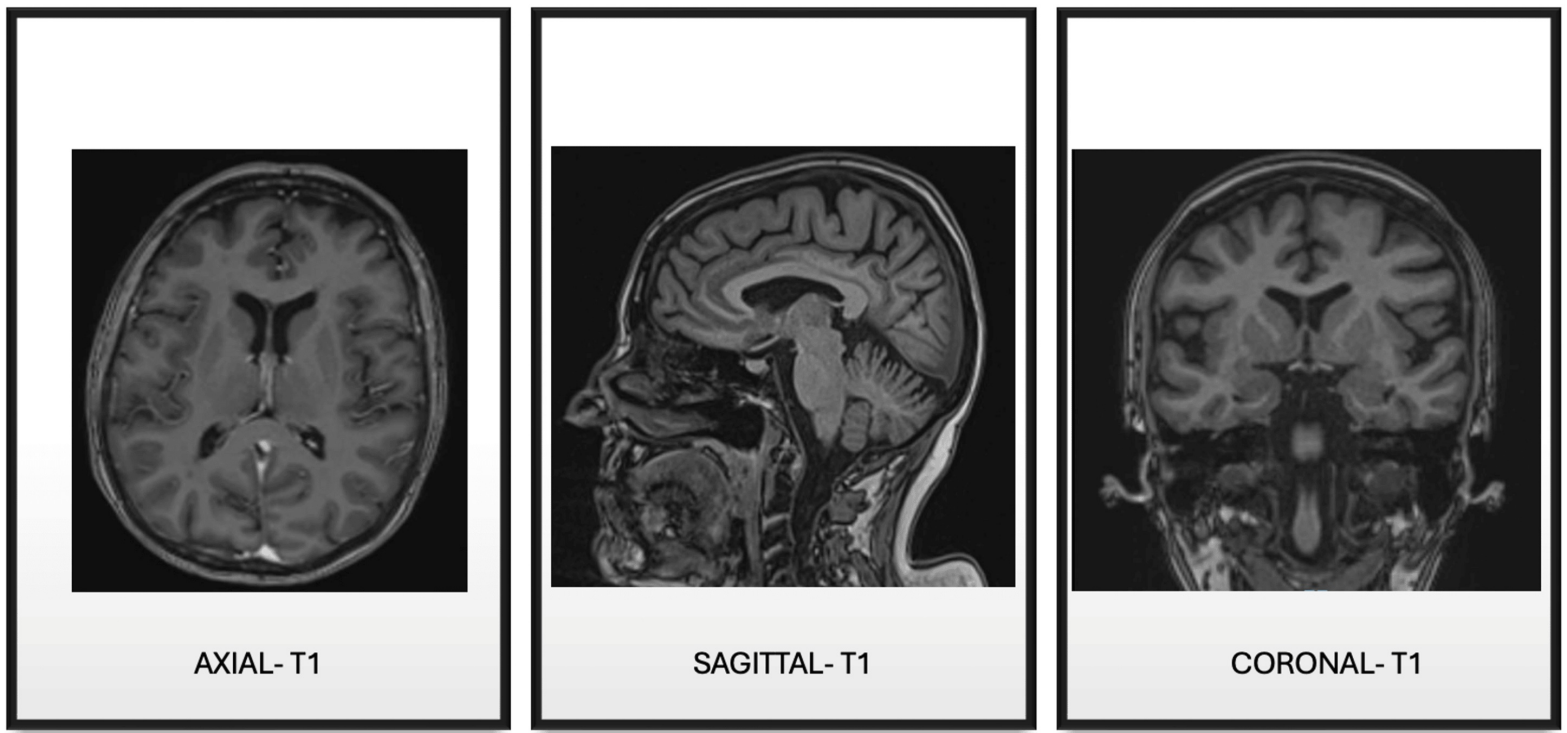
Brain magnetic resonance imaging. No significant age-related abnormalities were identified. No evidence of expansive, vascular, acute, or chronic lesions.
